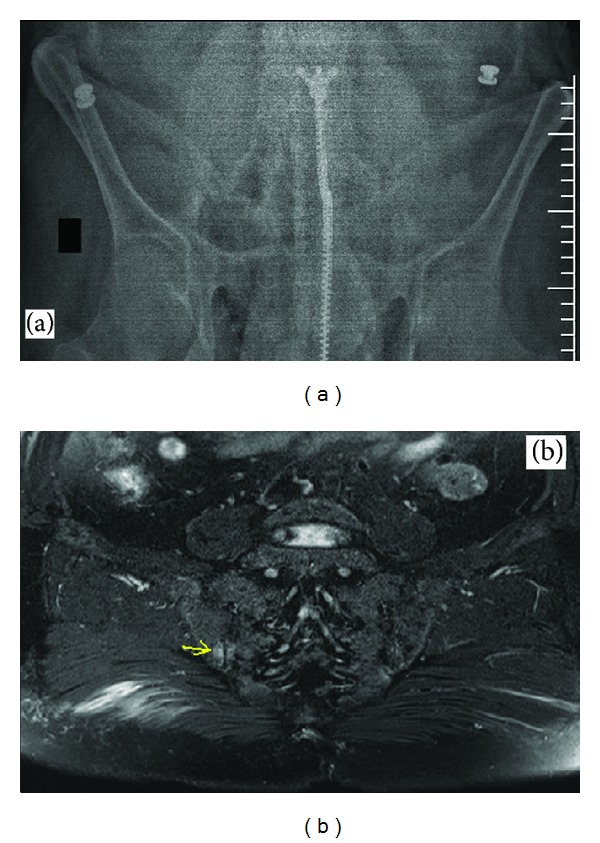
(a) Plain sacroiliac joint X-ray showing irregularities in each joint space (apparent in the right). (b) Evident bone marrow edema area in right side on sacroiliac magnetic resonance (MR) (yellow arrow).
